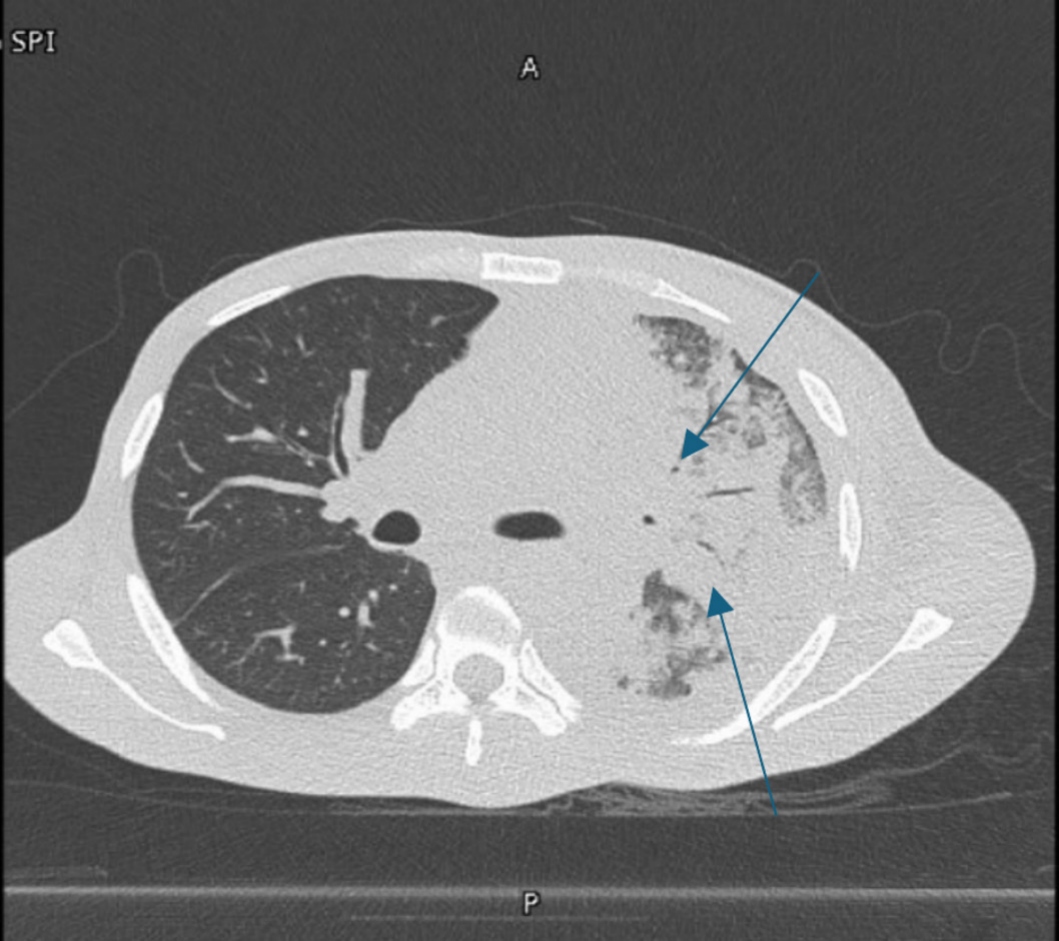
A contrast‐enhanced CT of the thorax showing a large malignant mediastinal mass invading the left brachiocephalic trunk blue arrows.
